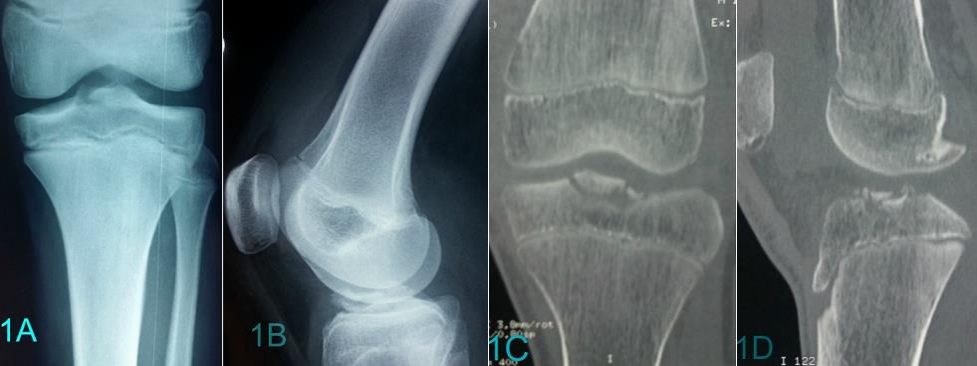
Cliché radiographique de face (A) et de profil (B) montrant une fracture des épines tibiales; (C, D) images tomodensitométriques avec reconstruction montrant la fracture des épines tibiales
